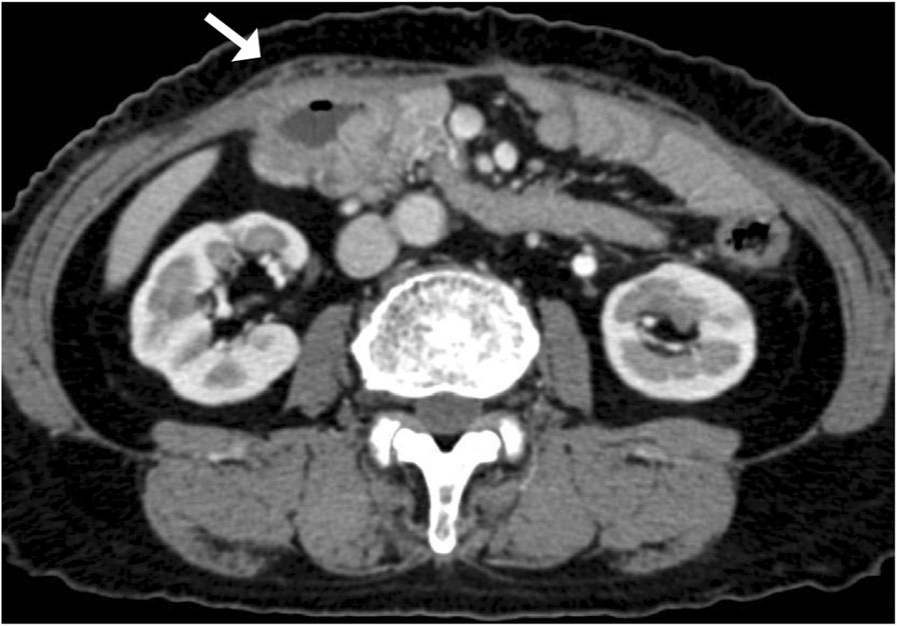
Contrast-enhanced abdominal CT scan showed wall thickness with enhancement in the second portion of the duodenum and the adjacent abdominal wall (arrow)
